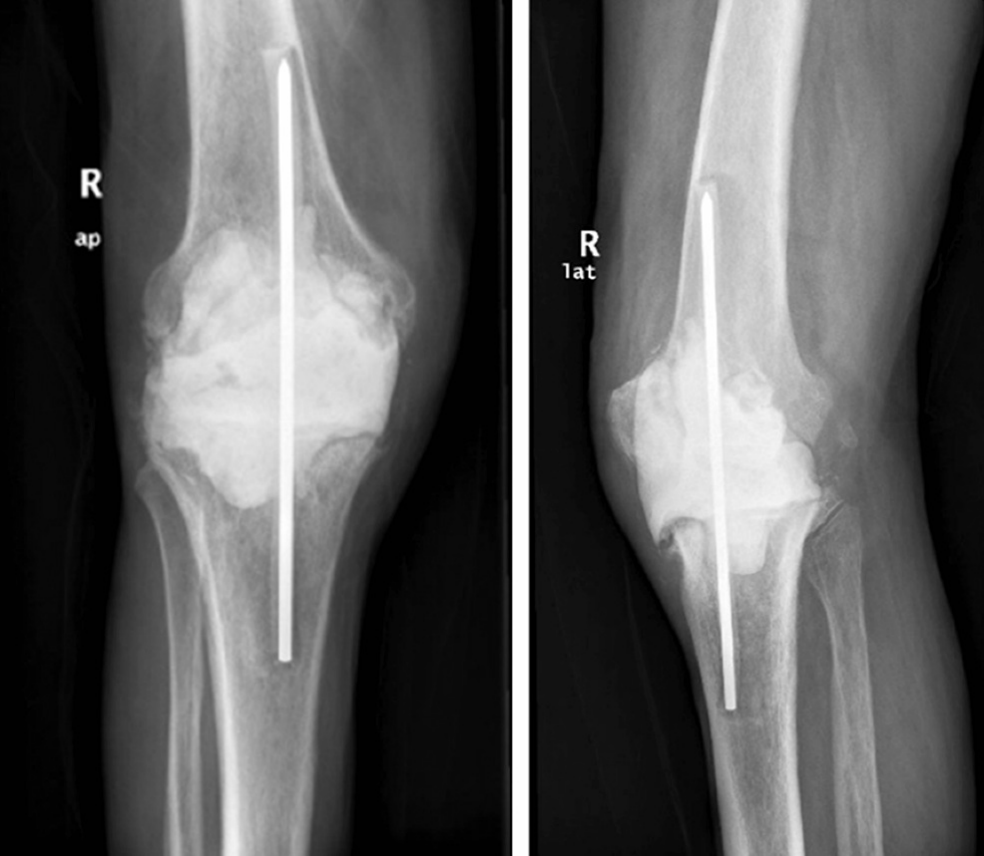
Postoperative radiographs after implant removal, joint arthrodesis using a Steinman pin and placement of an antibiotic-loaded bone cement spacer.
